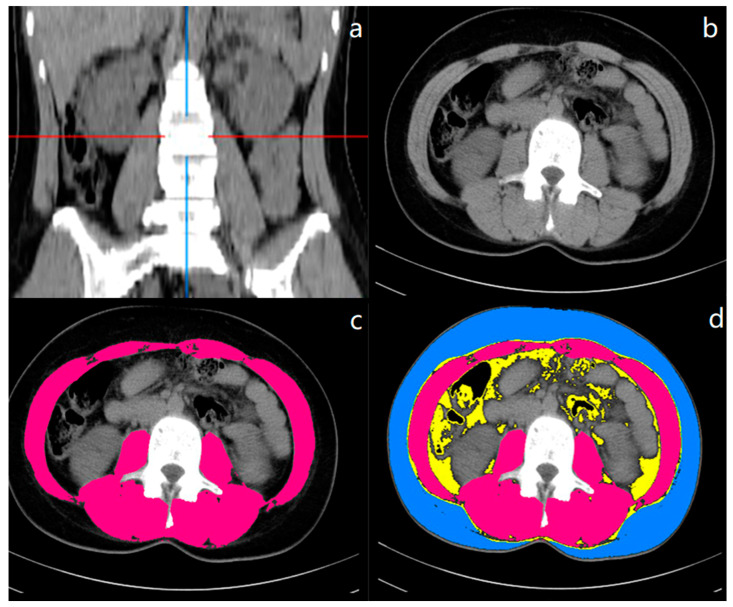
CT measurement of body composition at the level of the third lumbar vertebra (L3). (a) represented the coronal image and the red and blue lines identified the level of L3 and median coronal dividers, respectively. (b–d) represented the cross-sectional images. In the (c,d) images, different body composition areas were distinguished by different colors (blue: subcutaneous adipose tissue area; red: skeletal muscle area; the yellow area within the inner wall of the muscle: visceral adipose tissue area).
